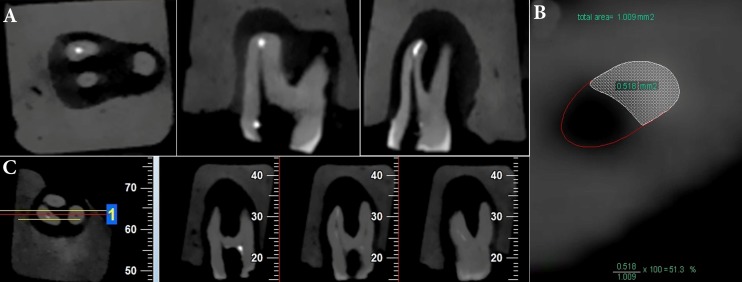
A)* Axial, coronal and sagittal CBCT cross-section; *B)* calculation of the surface areas; *C)* The cross-sections used for observational scoring
